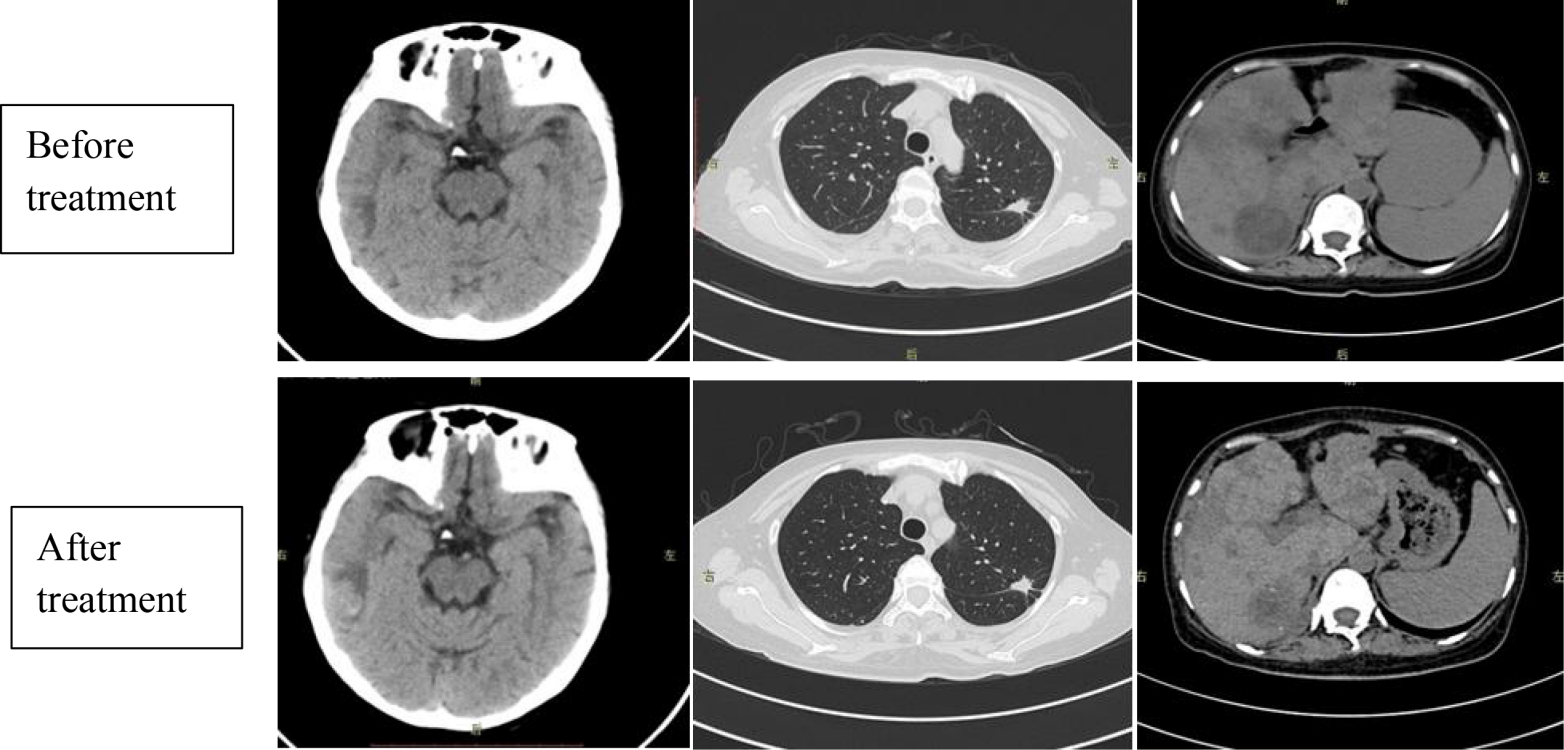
Comparative CT imaging before and after two cycles of treatment with olaparib, temozolomide, and atezolizumab.
